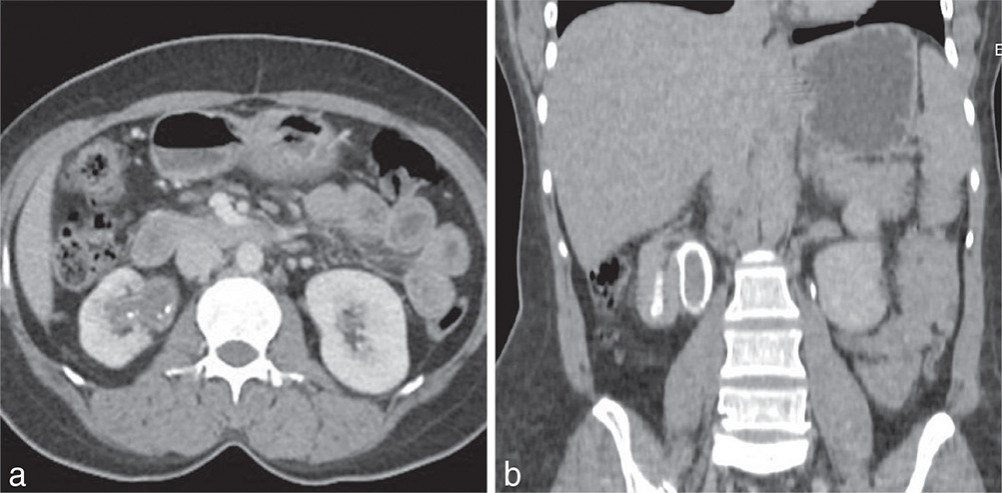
Contrast-enhanced CT images. (a) Nephrogram phase shows no enhancement of the lesion; subtle wall enhancement of the ureter is seen. (b) Delayed coronal reconstructed image shows contrast-filled urine all around the lesion.
